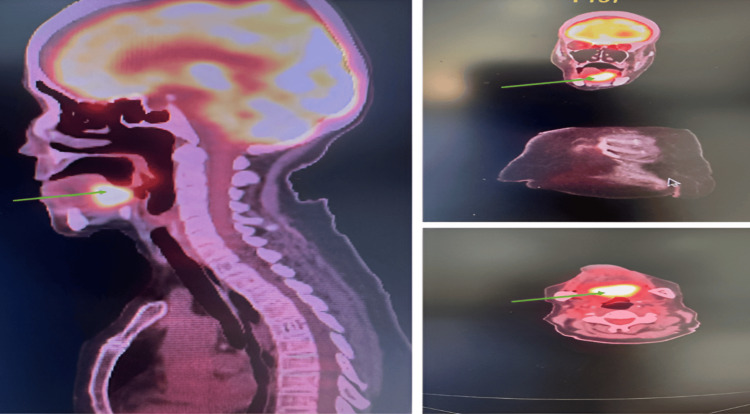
PET/CT images showing the hyperfixation process at the base of the tongue
